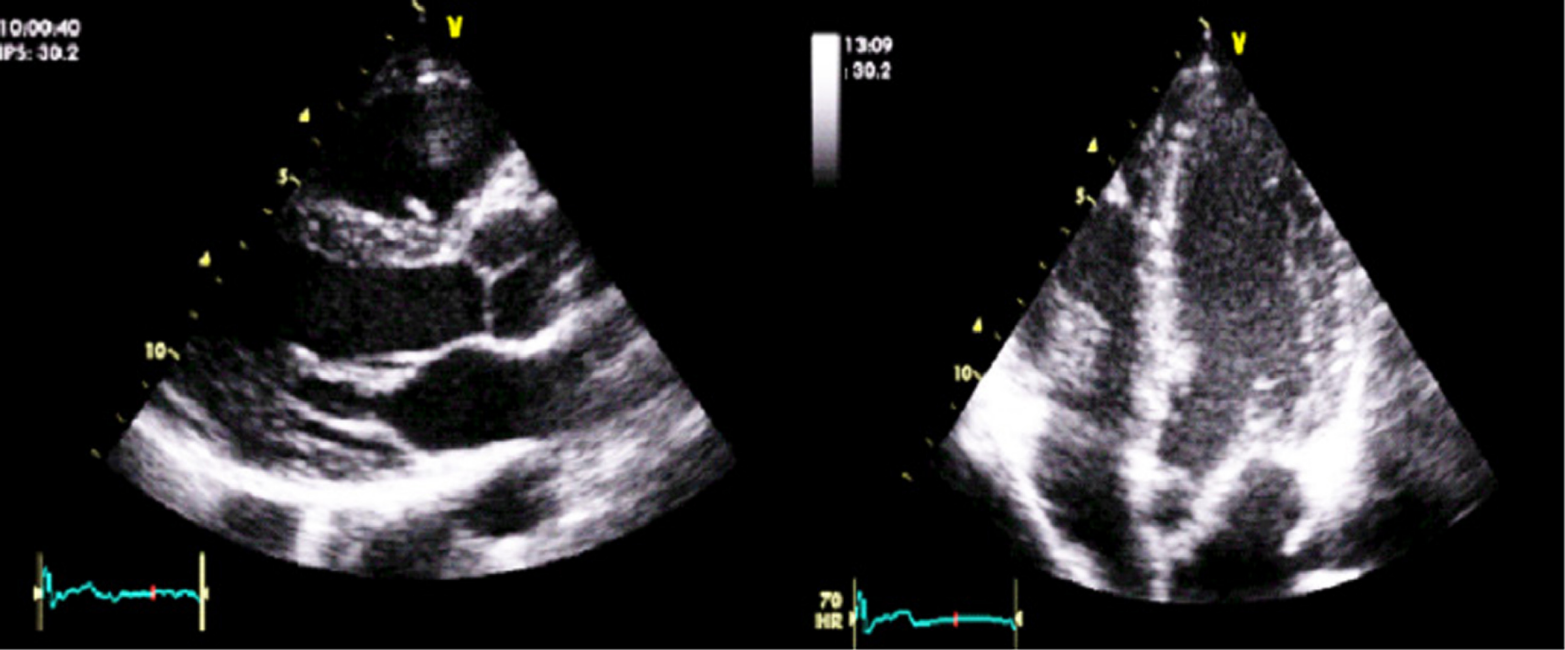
Echocardiogram findings indicated no significant structural heart disease.
